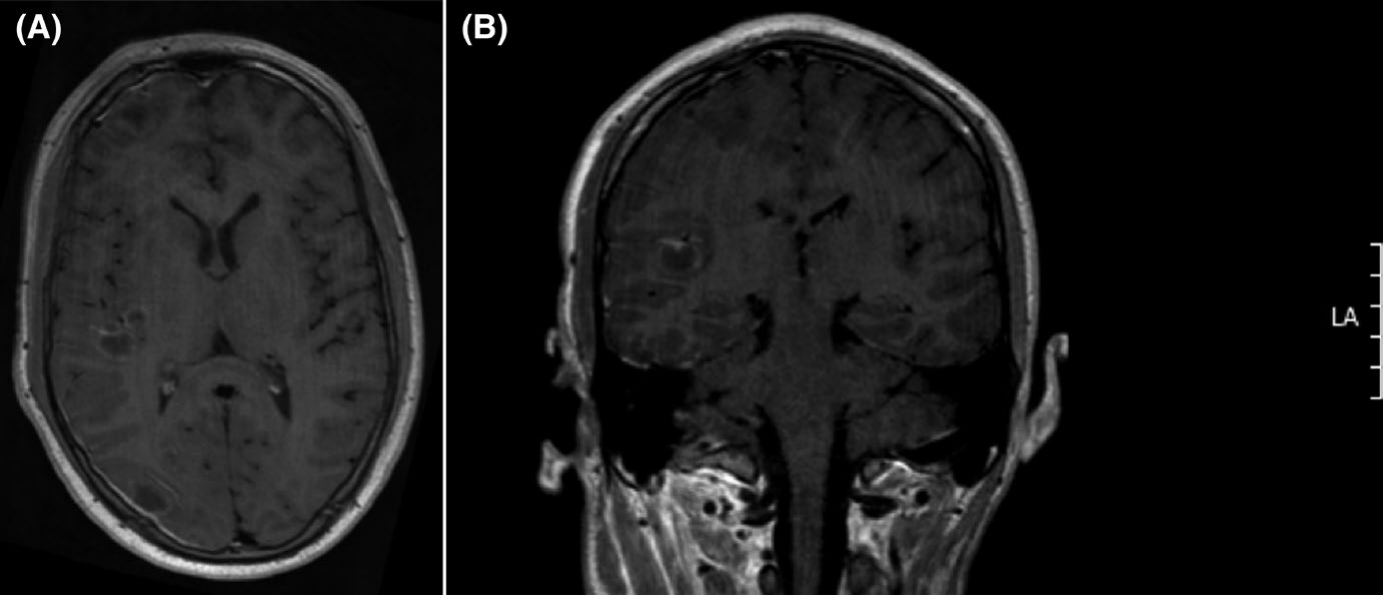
MRI Day two; axial (A) and coronal (B) T1‐weighted +contrast‐enhanced (CE) image demonstrating multiple intracranial abscesses
